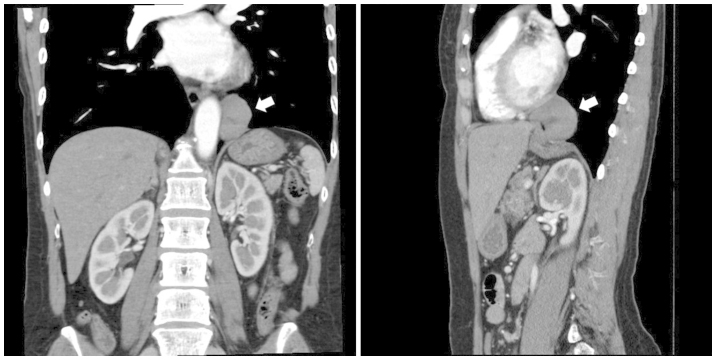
Computed tomography scan revealed a mass (arrow) at the distal esophagus.
